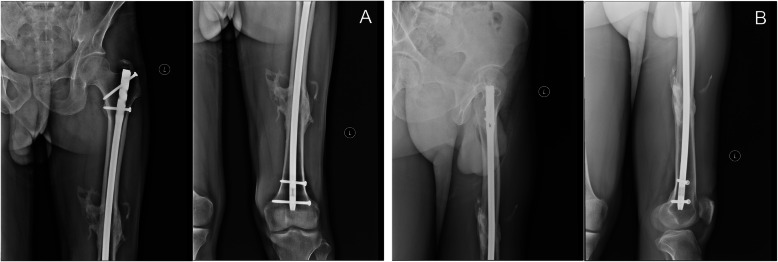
The view at 15 months postoperatively. a Anterior-posterior X-rays. b Lateral X-rays. Heterotopic ossification was observed
